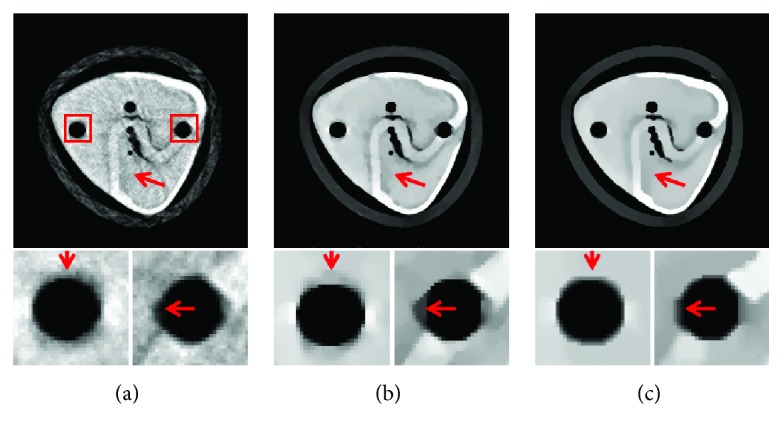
CT images of the modified buckwheat seed in experiment 1: (a) SART, (b) TV, and (c) TF-L0. The second row images are the close-ups of ROIs. The places pointed out by red arrows are examples where TF-L0 acquires the best results.
